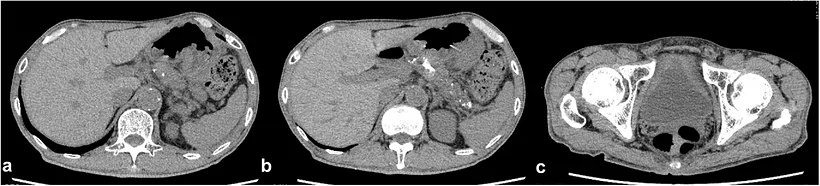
Follow‐up computed tomography after conservative management and pancreatic duct stent exchange. (a, b) Follow‐up computed tomography showing persistent diffuse pancreatic calcifications, consistent with advanced chronic pancreatitis. However, the peripancreatic fluid collections observed at the initial presentation had markedly decreased. (c) The pelvic encapsulated fluid collection had nearly disappeared. These findings suggested substantial improvement of pancreatitis‐related encapsulated peripancreatic and pelvic fluid collections after conservative treatment and maintenance of pancreatic duct drainage by stent exchange.
